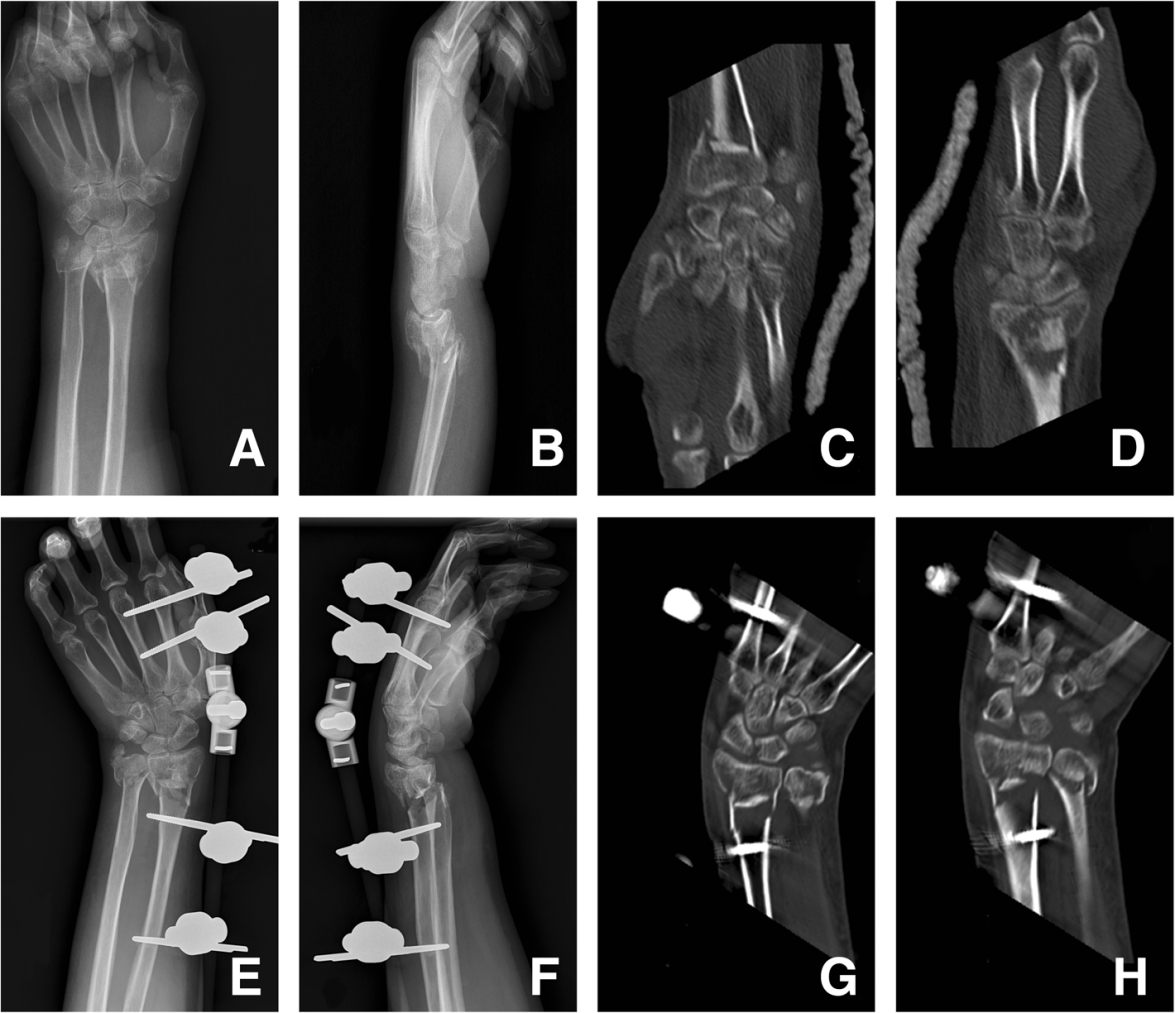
AO C3.1 fracture of the left distal radius occurred in a 52-year-old woman when she fell from standing height. a, b The preoperative posteroanterior and lateral X-ray. c, d CT scan showed the articular involvement with multi-fragments. e–h The good reduction and fixation by EF, from a view of X-ray and CT scanning
